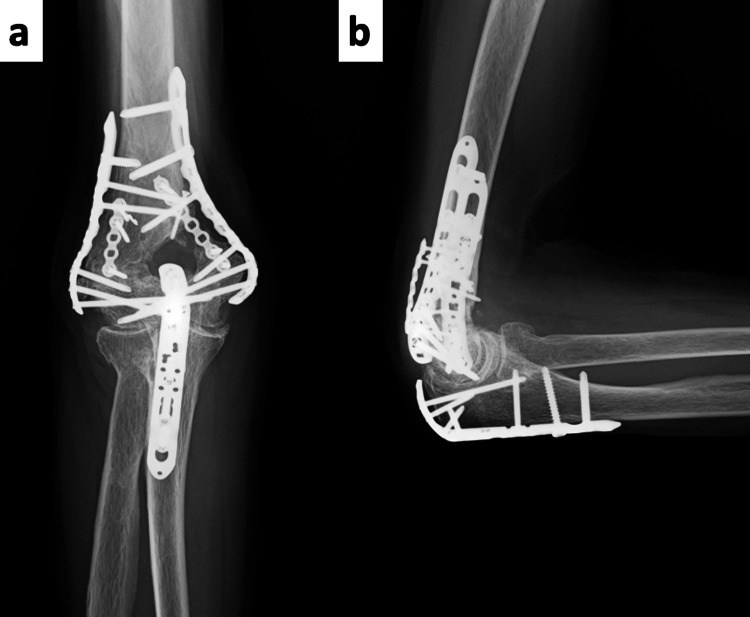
Final Follow-Up Plain Radiographs (a) Anteroposterior (AP) view; (b) lateral view. Bone union was confirmed, with no evidence of implant failure.
